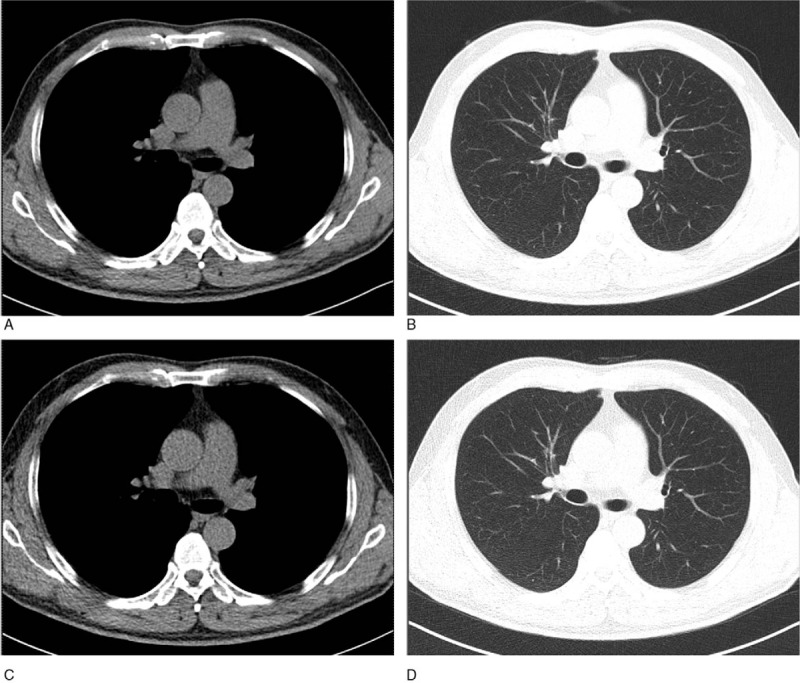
Transverse unenhanced multidetector images obtained with AP scout view (A and B) and AP-lateral scout view (C and D) shows roughly equal image quality on mediastinal (A and C) and lung (B and D) windows CT images, respectively. AP = anteroposterior, CT = computed tomography.
